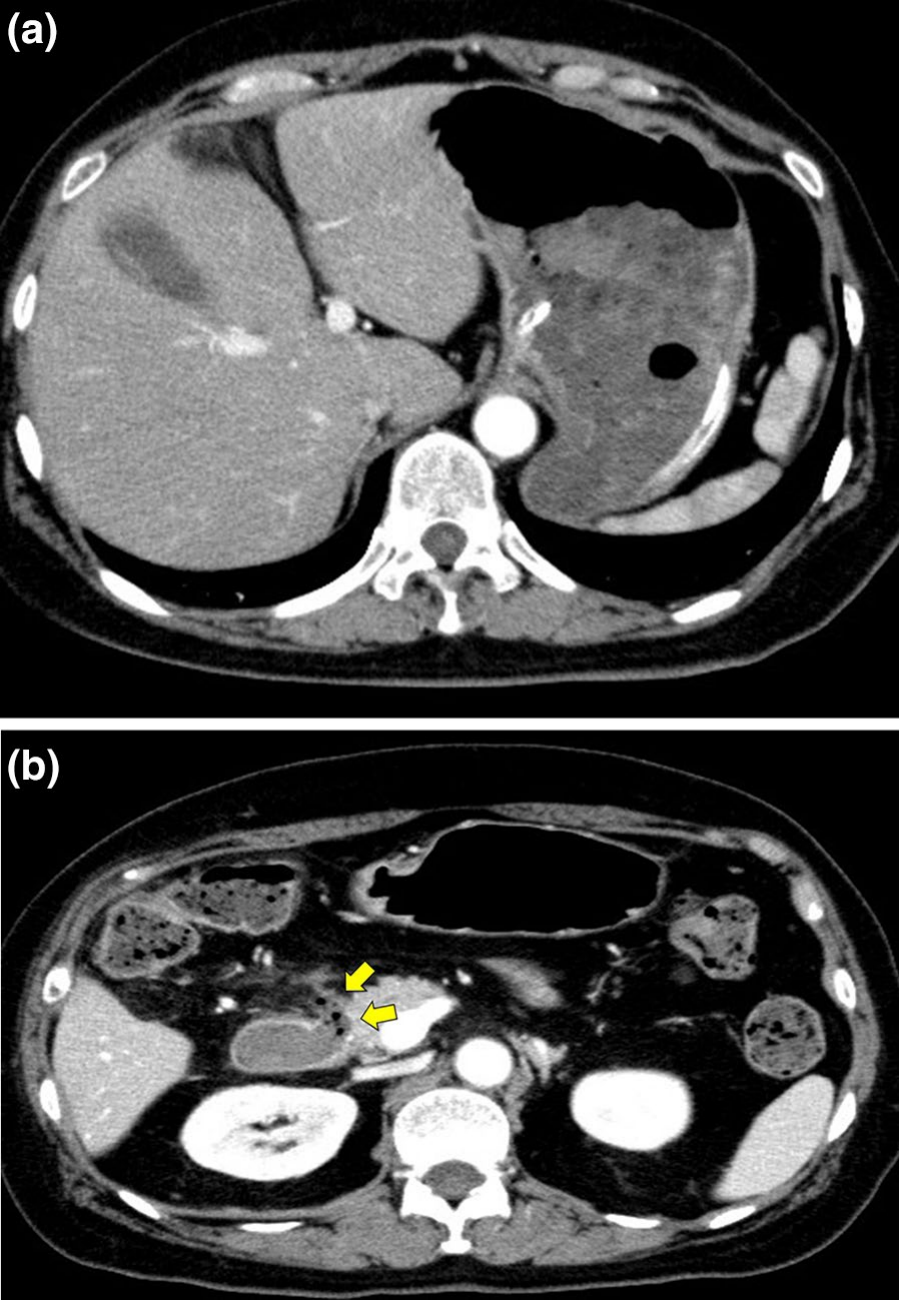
Contrast-enhanced CT. a Contrast-enhanced CT of the abdomen shows a large amount of hemorrhage in the stomach, suggesting massive gastrointestinal bleeding. b Free air is observed near the duodenal bulb (arrow)
